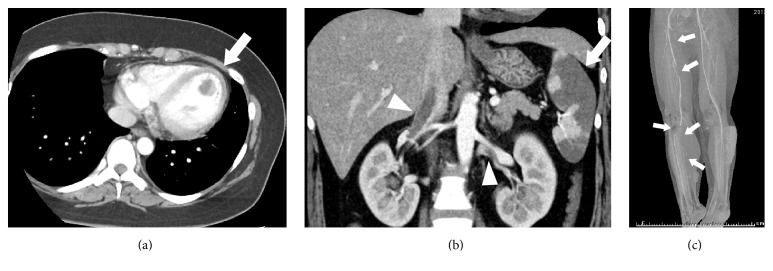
CT findings. (a) A ball-like thrombus located in the left ventricle (arrow). (b) Splenic infarction (arrow) and thrombi in bilateral renal veins and the inferior vena cava (arrowheads). (c) 3D reconstruction image of the lower limbs showing defects in the right superficial femoral artery, right deep femoral artery, right popliteal artery, right posterior tibial artery, and right peroneal artery (arrows).
